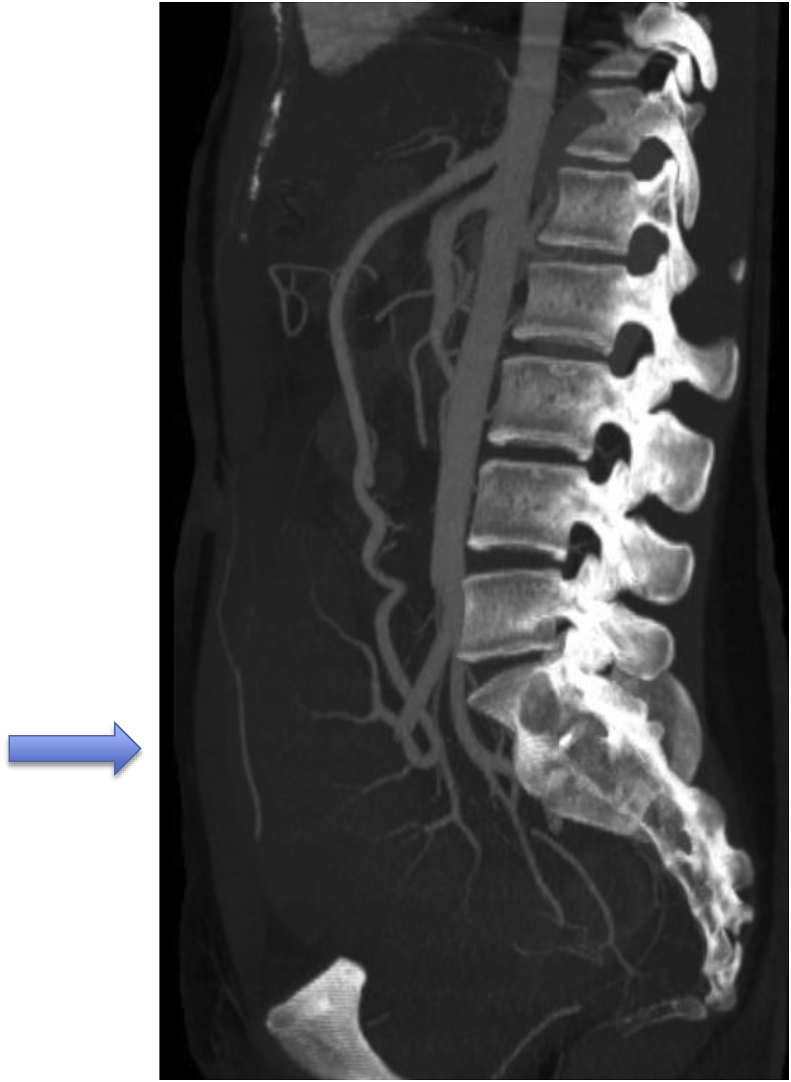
Axial contrast-enhanced CT image, MPR reconstructions, shows the splenic hilum.
